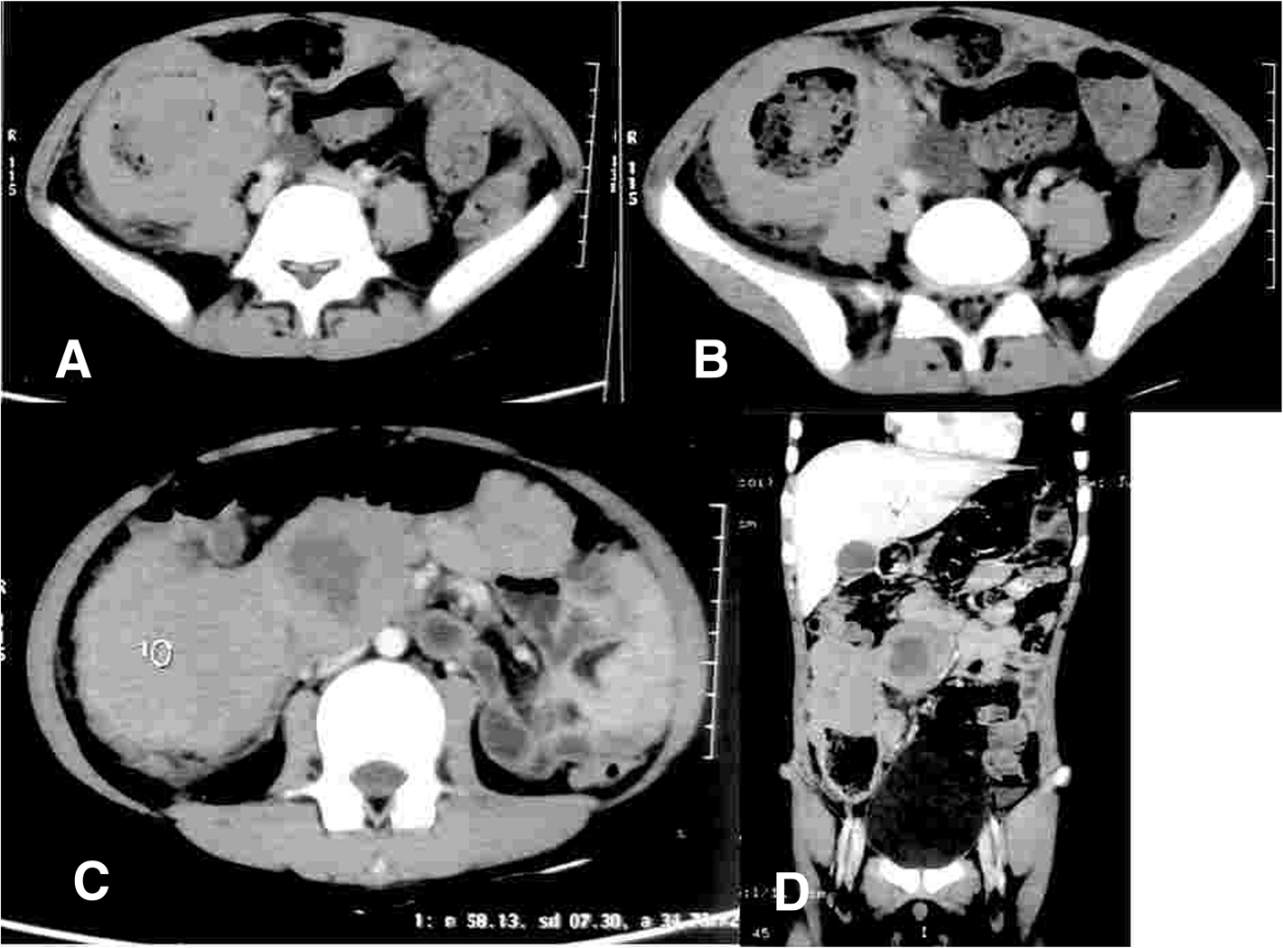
Computed tomography showing a) the lesion in the caecum, b) lesion in caecum with lymph nodes c) ascending colon and d) reaching up to transverse colon
